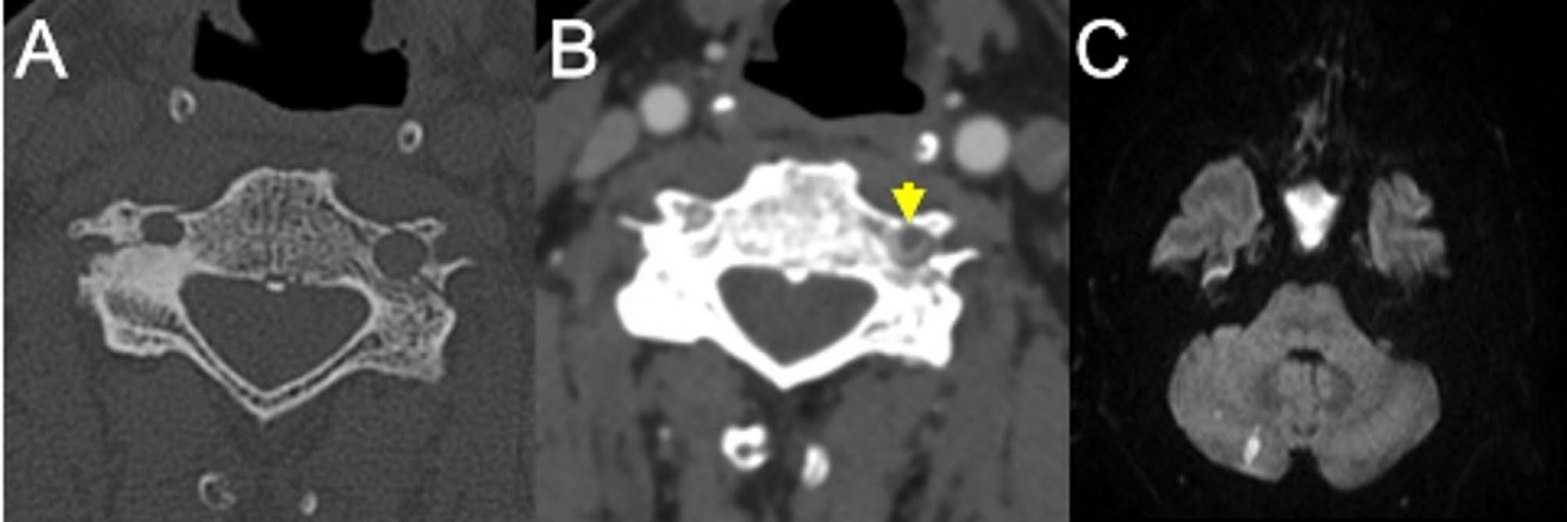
Post-traumatic cervical CT, CTA and MRI of a patient in the sixth decade of life involved in a car accident. Non-enhanced CT scan shows bilateral fractures of the lateral masses of a cervical vertebra (A), with involvement of the transverse foramina. CTA (B) shows partial lack of opacification of the left vertebral artery, consistent with post-traumatic arterial dissection (arrow) (Denver grade II). The MRI (C) shows foci of restricted diffusion in the diffusion weighted sequence in the right cerebellum, consistent with acute ischemic lesions of embolic origin from the left vertebral artery
